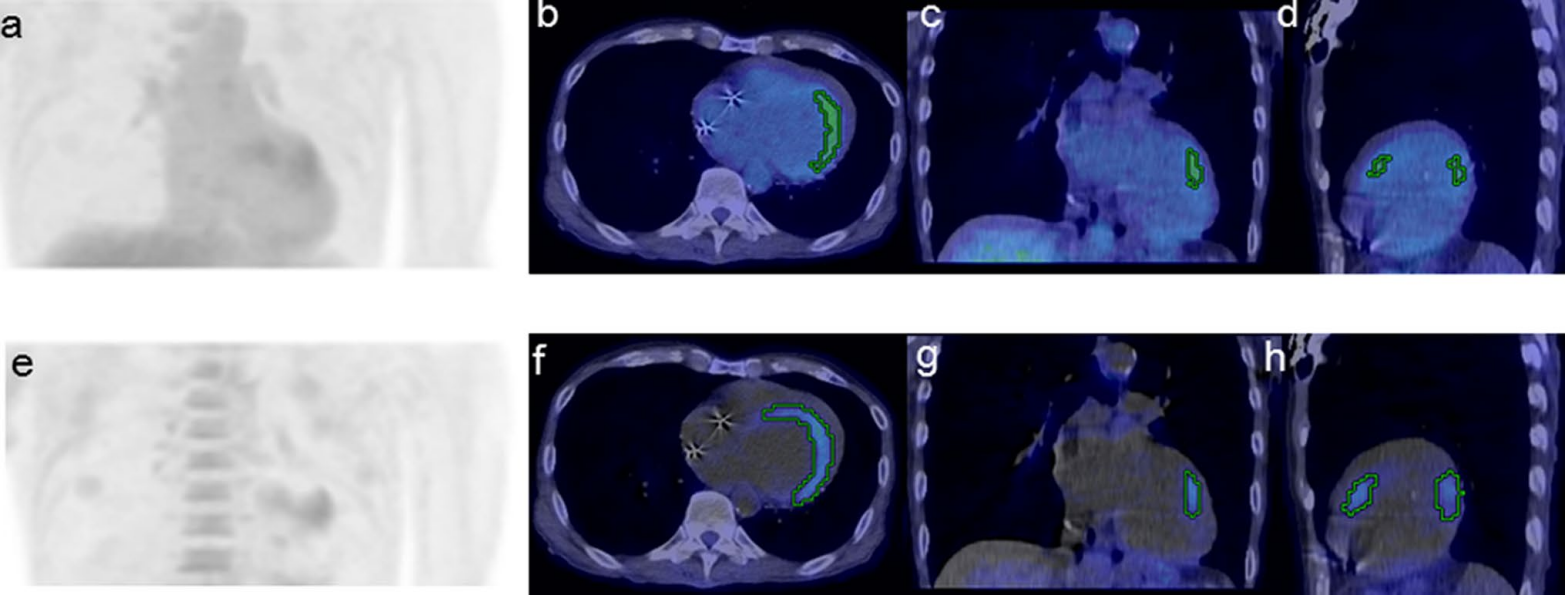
A 68-year-old male patient with CS who showed VT and cardiac dysfunction (EF: 25.3%) under steroid treatment (prednisolone, 10 mg daily). The SUV images [MIP (a), transaxial (b), coronal (c), and sagittal (d)] and Ki images [MIP (e), transaxial (f), coronal (g), and sagittal (h)] both show the positive myocardium for which the VOIs were automatically determined. The Ki volume (43.3 cm^3^) was the only parameter positive for the risk of arrhythmic events according to each threshold criterion.
